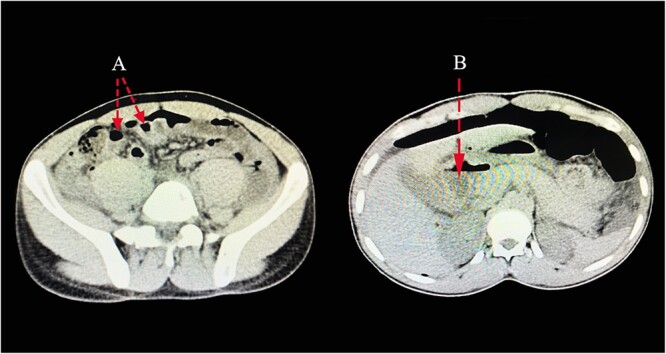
CT scan images pointing out presence of pneumoperitoneum related to a perforation (A), with free fluid collection in the abdomen (B).
